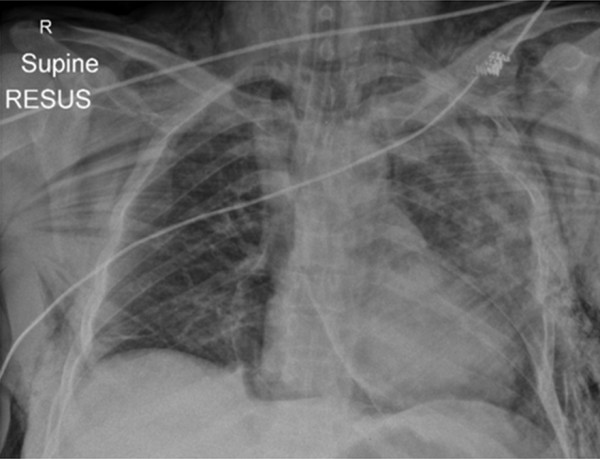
Plain supine AP chest radiograph showing extensive surgical emphysema; multitude of rib fractures and flail on the left side; bilateral pulmonary contusions and suggestion of a haemothorax on the left side; a rotated 'boot shaped' cardiac silhouette, with clear demarcation of cardiac silhouette from the diaphragm; pneumomediastinum; the pericardial contour is also distinctly visible; endotracheal tube.
